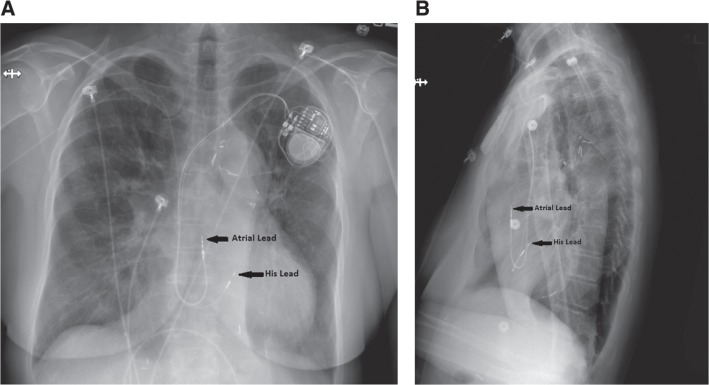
A: Anteroposterior chest radiograph showing lead placement in a His-bundle pacing system in a patient with a structurally normal heart. B: Lateral chest radiograph showing lead placement in a His-bundle pacing system in a patient with a structurally normal heart.
